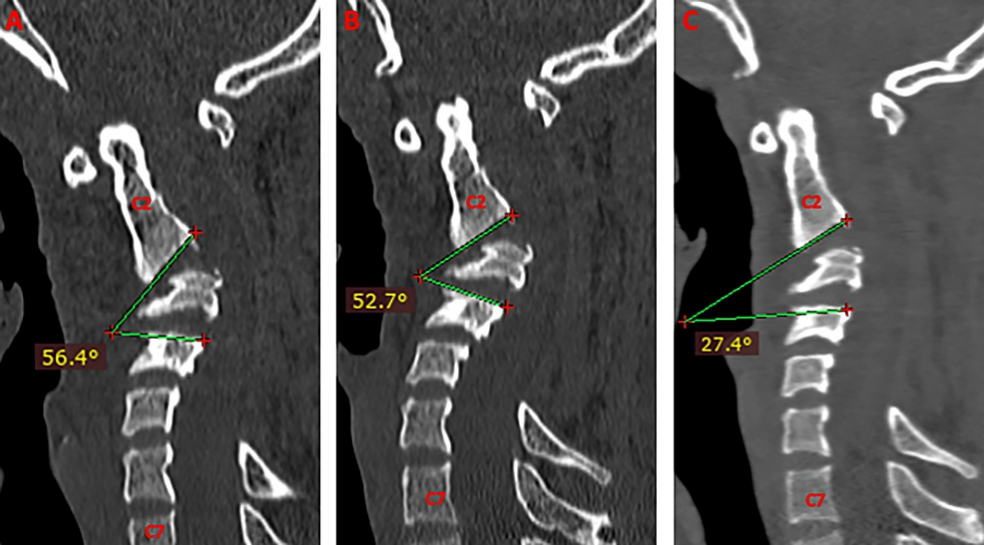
CT sagittal view showing a gradual reduction in the local kyphosis/Cobb’s angle with a gradual increment in the traction weights. (A) 3 Kg; local Cobb’s angle of 56.4 degrees. (B) 5 kg; local Cobb’s angle of 52.7 degrees. (C) 8 kg; local Cobb’s angle of 27.4 degrees.
